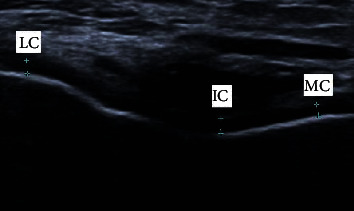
Transversal ultrasound suprapatellar scan of the knee joint in right femoral cartilage; lateral condyle (LC), intercondylar (IC), and medial condyle (MC).
